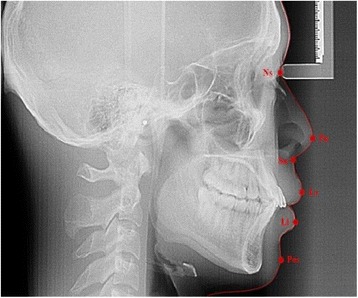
Cephalometric radiograph showing soft tissue landmarks.
